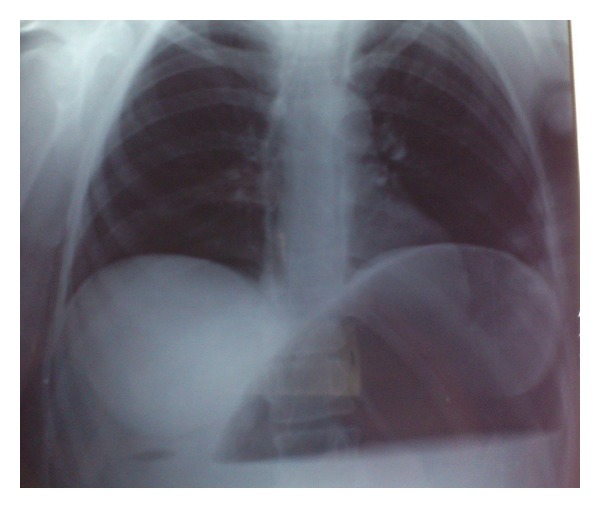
Direct abdominal X-ray image, obtained in a standing position, showing air almost completely filling the left upper quadrant of the fundus and extending into the right upper quadrant.
